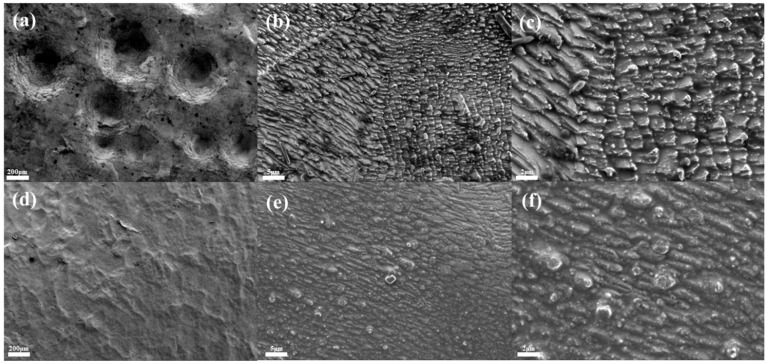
SEM images of tin sheet surfaces after ultrasonic treatment (a–c) and after conventional treatment (d–f).
